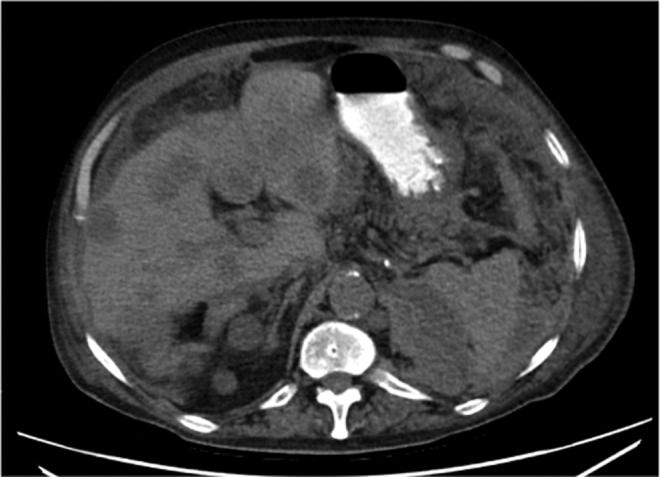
Case 1: CT scan from January 2014 following left nephroureterectomy demonstrating diffuse new hypoattenuating lesions consistent with metastatic disease. There is also peritoneal carcinomatosis, increased lymphadenopathy, and moderate intra-abdominal ascites.
